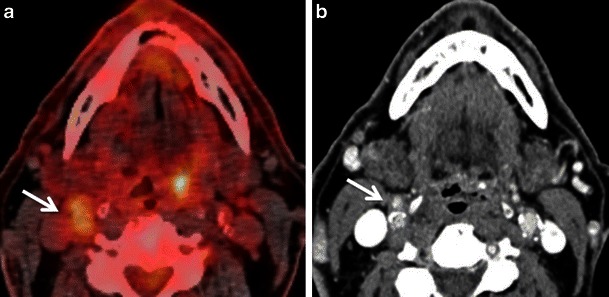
a Axial PET/CT image depicts a focal nodular area of high FDG uptake at right level II (*arrow*) and at the left base of tongue (*arrowhead*). This patient was a follow-up case of SCCof the right floor of mouth. The nodular FDG uptake at right level II was considered suspicious for nodal recurrence whilst the focal FDG uptake at the left base of tongue was ascribed to probable lymphoid tissue uptake. b Corresponding axial CECT image detects no evidence of metastatic cervical adenopathy at right level II. The focal FDG uptake at right level II corresponds to partly calcified plaques in the right internal and external carotid arteries (*arrow*). Follow-up confirmed no lesion in the left base of the tongue
